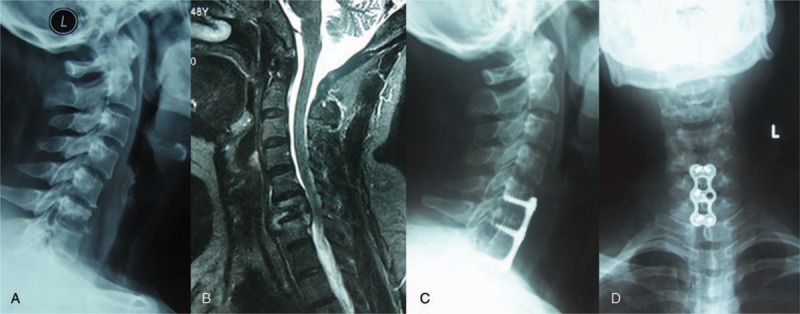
Female, 45 y, C6–7 tuberculosis: x-ray and MRI showed the destruction of vertebral bodies of C6–7 (A and B). The lateral and anteroposterior view of x-ray showed that the anterior infected site had healed and bony union was achieved at the final follow-up (C and D). MRI = magnetic resonance imaging.
